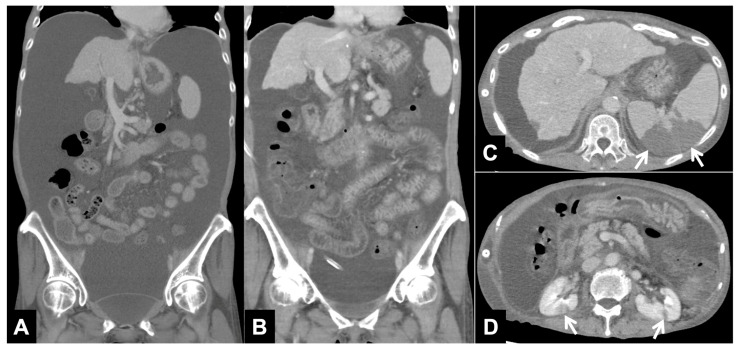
CT images with continuous elevation of D-dimer (Patient No. 12 in Table 2). (A) Reconstructed coronal CT image before PVS placement shows a large amount of ascites. (B) Reconstructed coronal CT image one week after PVS placement shows a decrease in ascites. (C,D) Axial CT images one week after PVS placement shows splenic infarction ((C) arrows) and bilateral renal infarction ((D) arrows).
